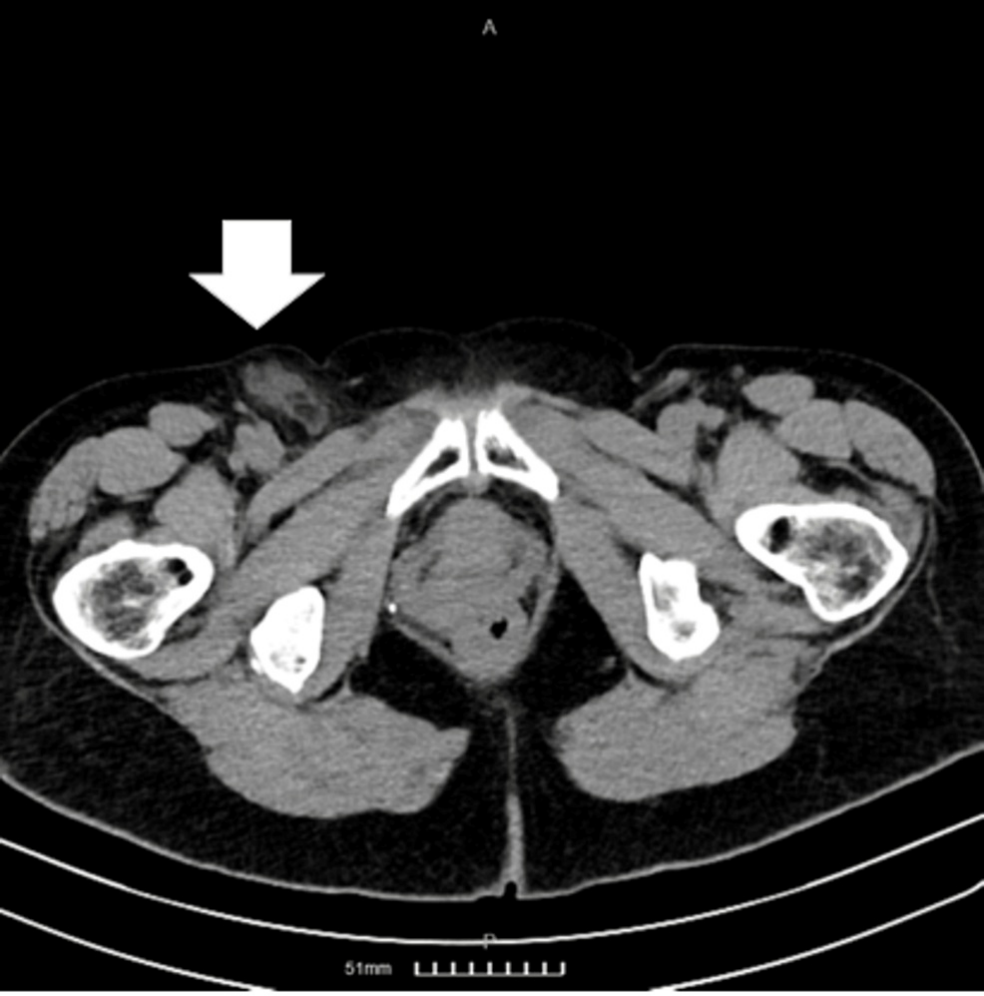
An axial view of the CT showing a femoral hernia containing the appendix
